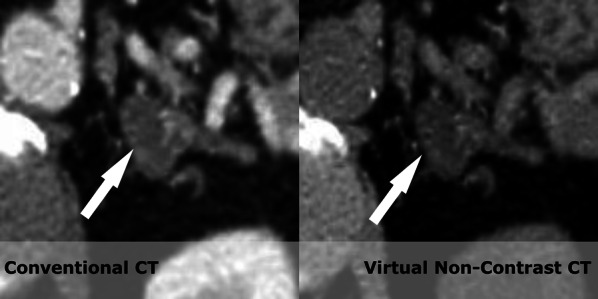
A lesion (white arrow) in the left adrenal is seen with HU values of 46 on the conventional portal venous phase scan. On the virtual non contrast series HU value of 2 was measured. As no malignant findings were made in the patient the correct diagnosis of an adenoma was made. This was confirmed by a true non contrast CT examination
